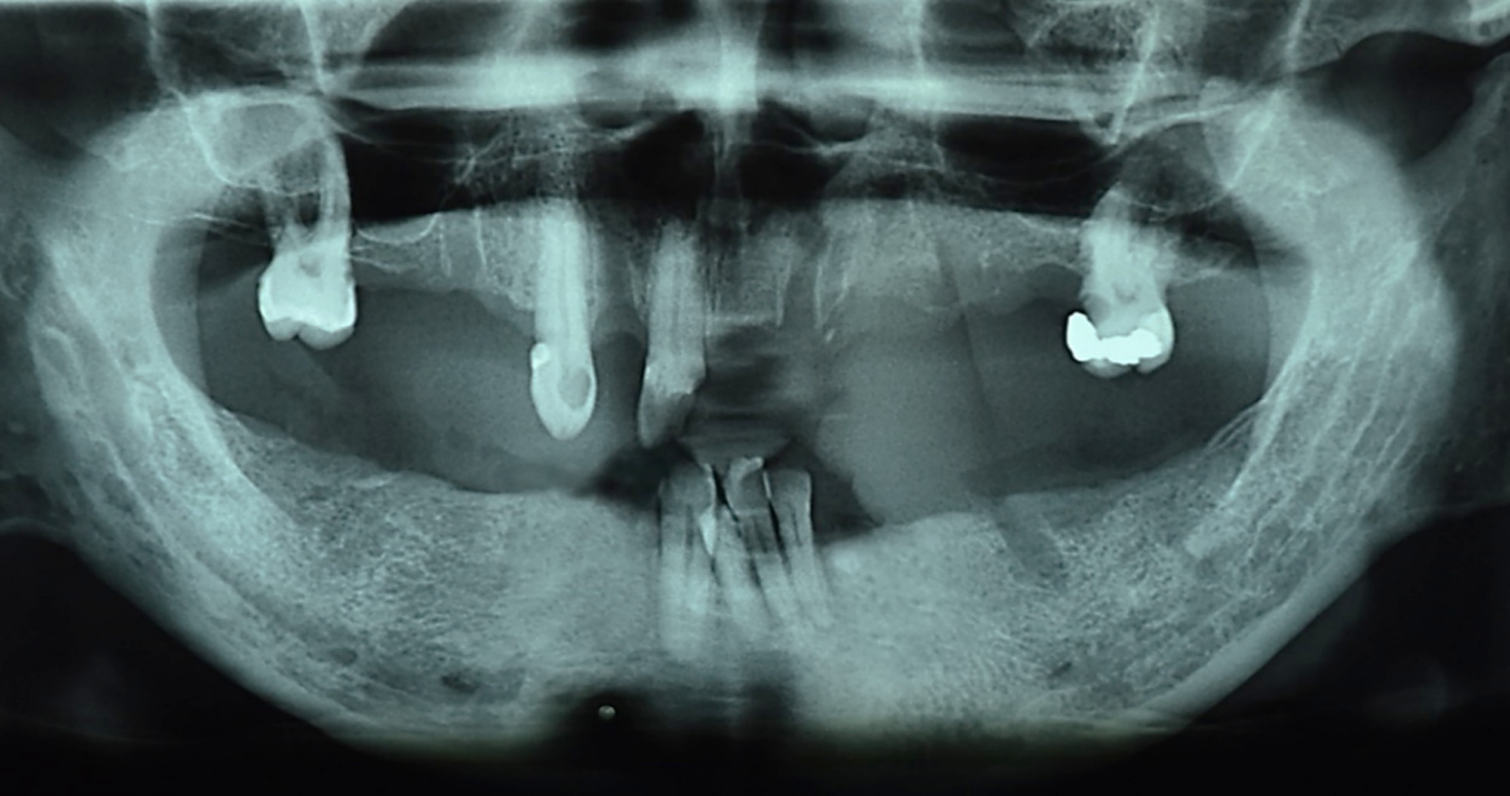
Dental panoramic radiograph
